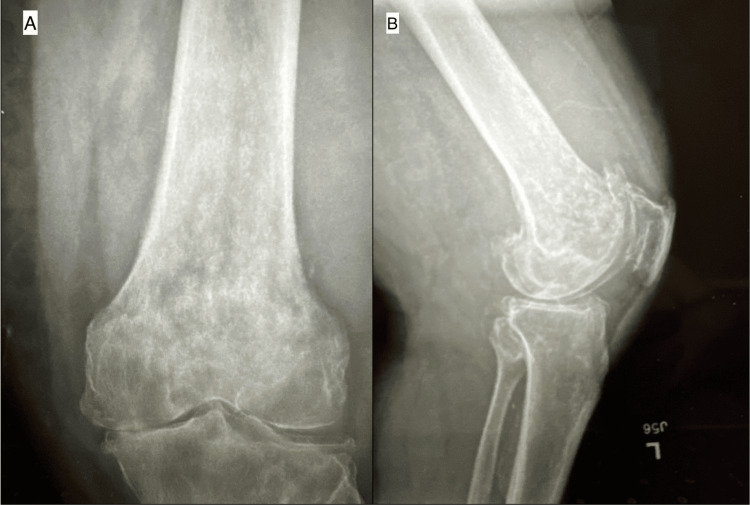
Preoperative (A) anteroposterior and (B) lateral knee radiographs.
